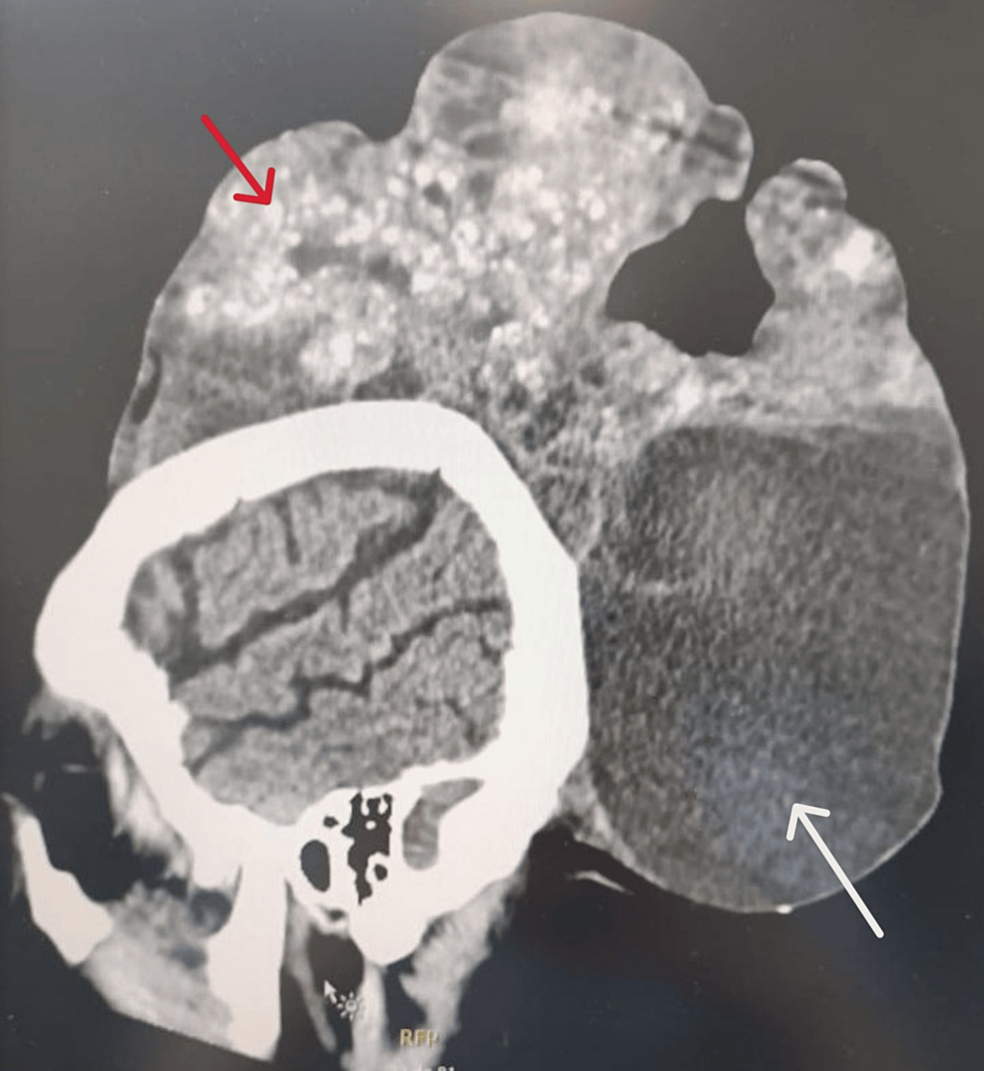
Lateral view of the patient's head on the CT scan, which showed a lesion of 21 cm in transverse axis, 16.5 cm in longitudinal axis, and 16 cm in anteroposterior axis. A cystic formation (white arrow) can be noted, as well as some calcified areas on the lesion (red arrow). CT: computed tomography
